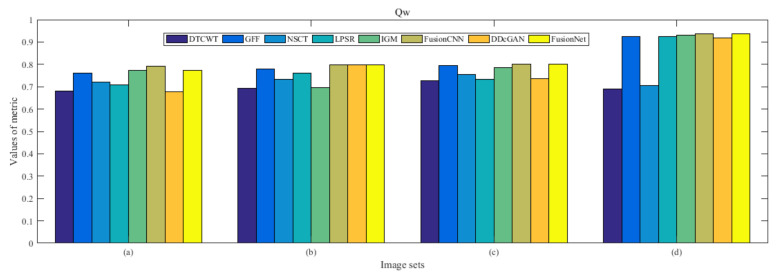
Values of $Q_{w}$ in the fused images (MRI-FDG): (a) is composed of eight images in Figure 25, (b) is composed of eight images in Figure 26, (c) is composed of eight images in Figure 27, (d) is composed of eight images in Figure 28.
